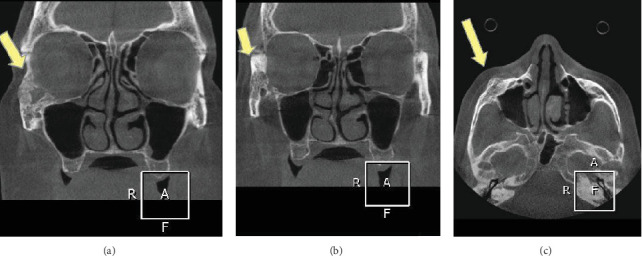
(a, b) Coronal CBCT scan and (c) axial CBCT scan of central hemangioma in the zygomatic bone.
